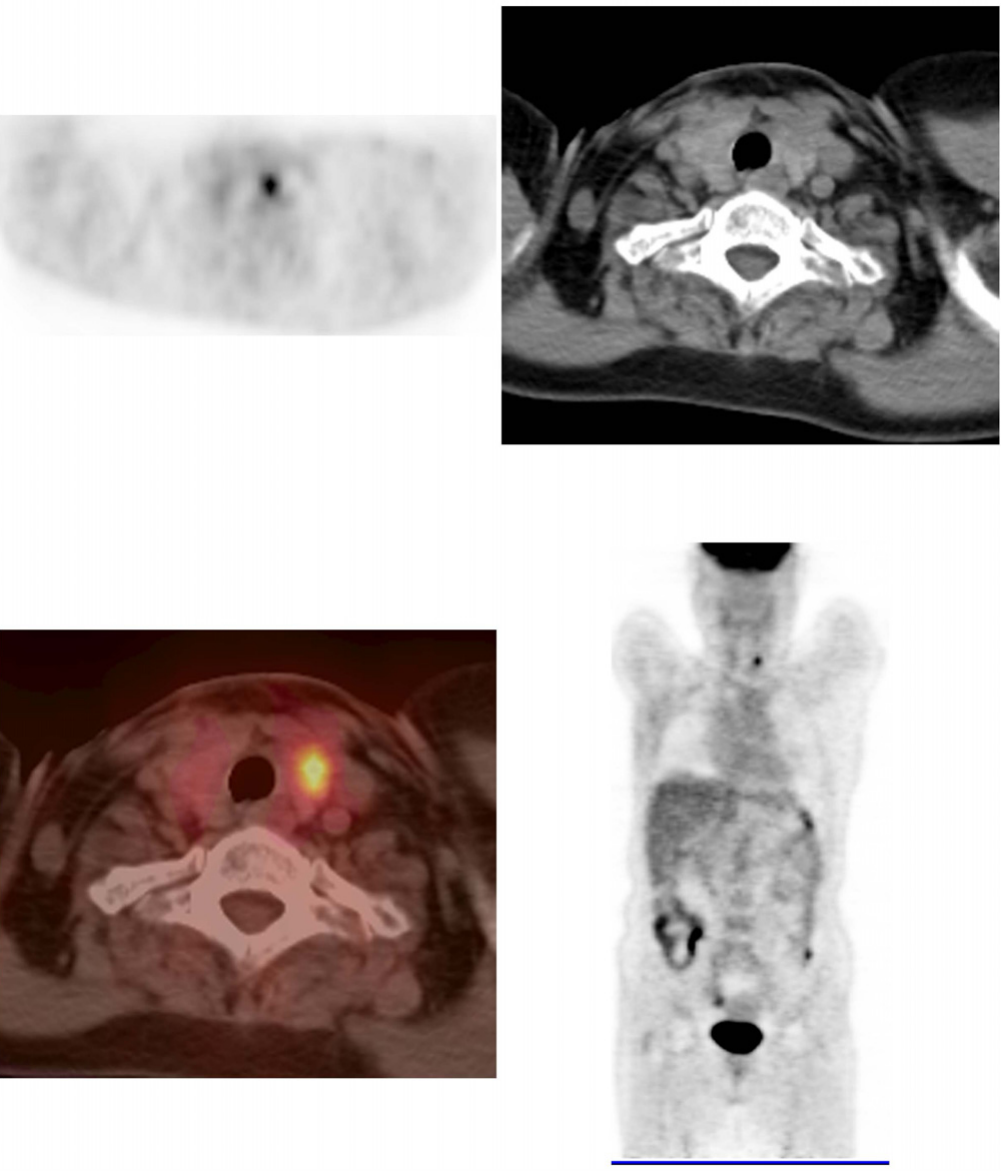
Focal FDG uptake on PET/CT. 54 year old female with breast cancer. The ^18^F-FDG PET/CT revealed focal uptake with SUV of 7.6. The patients was performed total thyroidectomy with a final diagnosis of papillary thyroid carcinoma.
